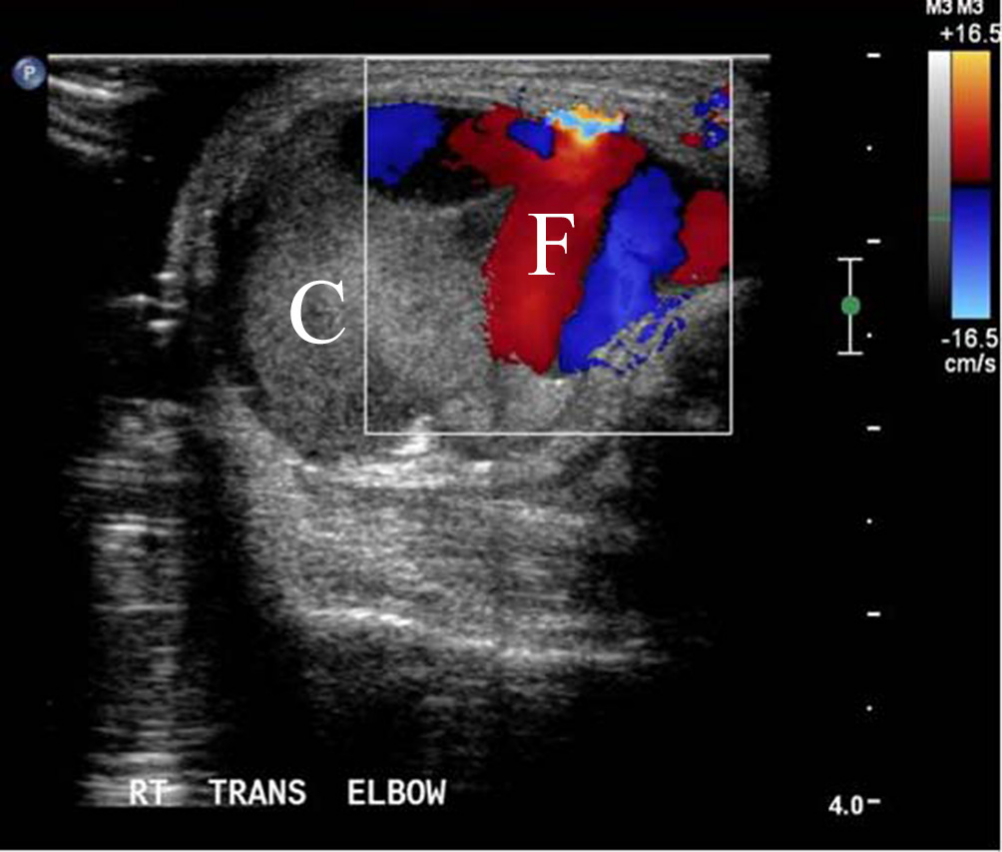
Colour Doppler study performed 24 h after the initial thrombin injection showing partial reopening of the lesion: colour Doppler flow (F) around the clot (C).
